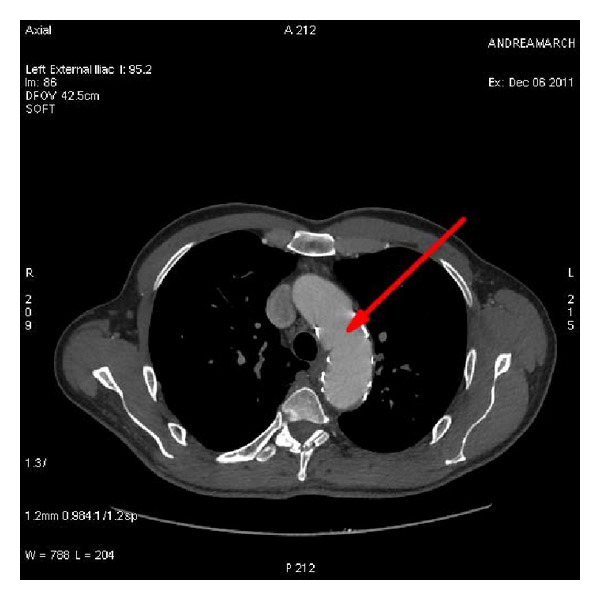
Six-month follow-up CTA shows the thoracic aorta without false lumen.
